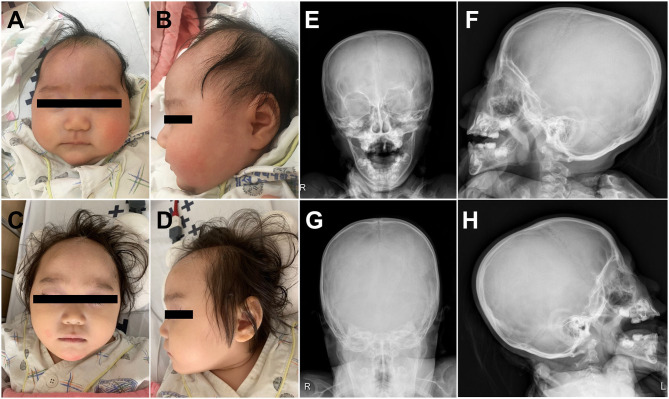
Clinical photographs of the proband showed microcephaly without craniofrontonasal dysmorphism at age of 4 months (A,B) and at age of 2 years (C,D). Skull X-ray of the proband revealed no craniosynostosis (E–H).
